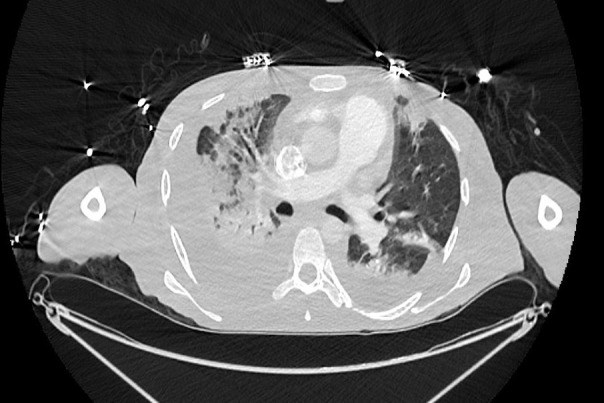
Initial CT scan of the thorax following endotracheal intubation showing extensive pulmonary metastases and pneumonia.
